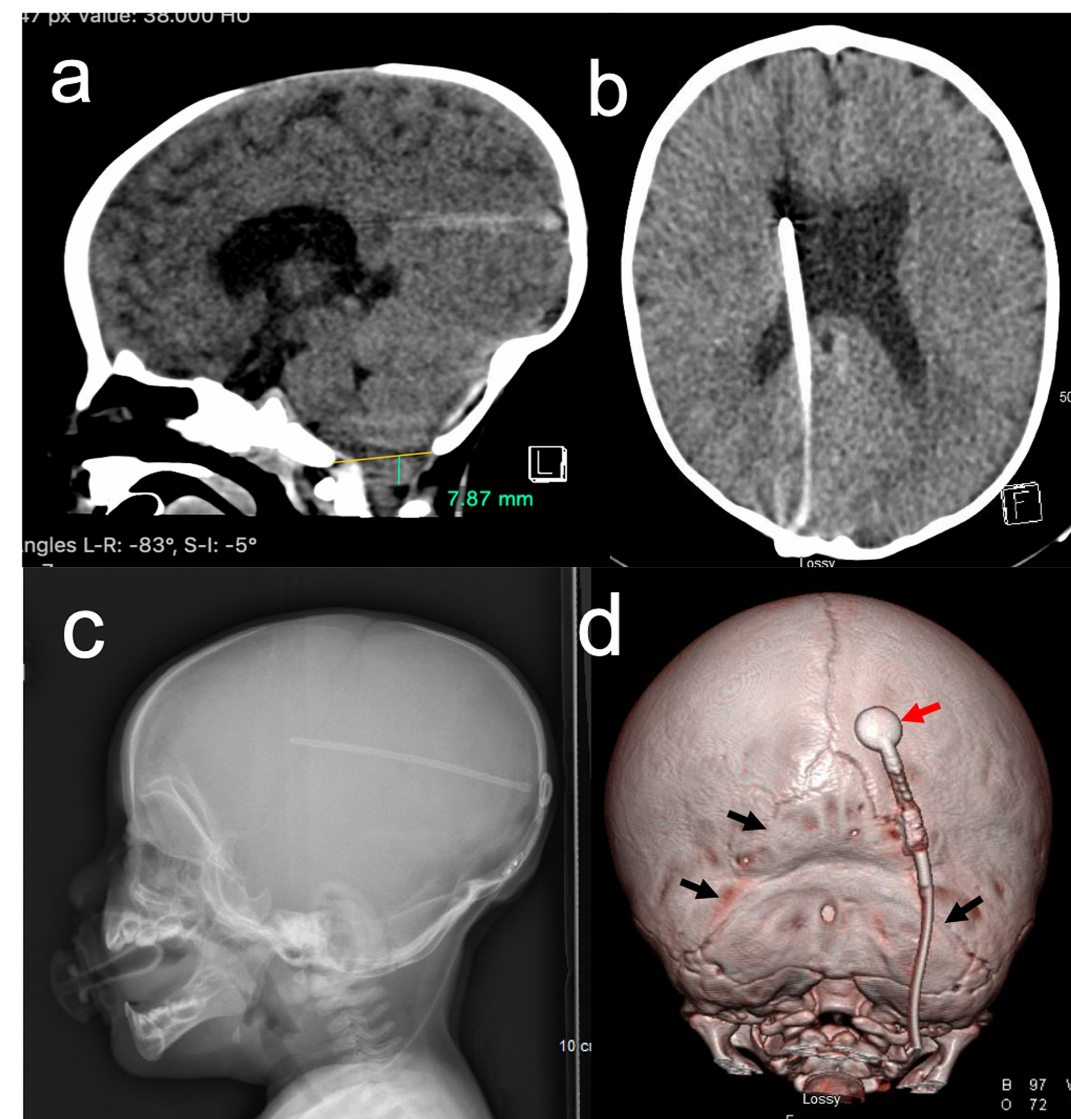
Preoperative CT and x-ray Preoperative axial CT image (a and b), x-ray (c) and 3D modeling (d) demonstrate bilateral lambdoid synostosis with flattening and deformation of the occipital bone and restriction at the level of the foramen magnum. Panel (a) demonstrates tonsillar herniation of 7.87 mm (green) below McRae line (in yellow). Shunt hardware is present at the traditional parieto-occipital entry point with valve (red arrow) and distal tubing overlying the operative area of interest (black arrows) for synostosis surgery.
